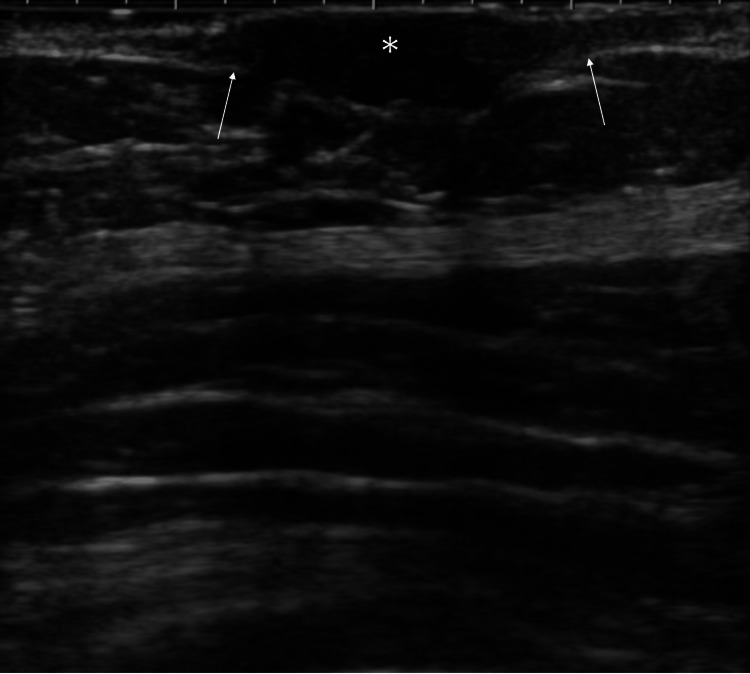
Ultrasound findings Ultrasound showed a mass in the areolar skin (asterisk) which had a hypoechoic pattern, unchanged posterior echoes, and disruption of the dermis (arrows)
